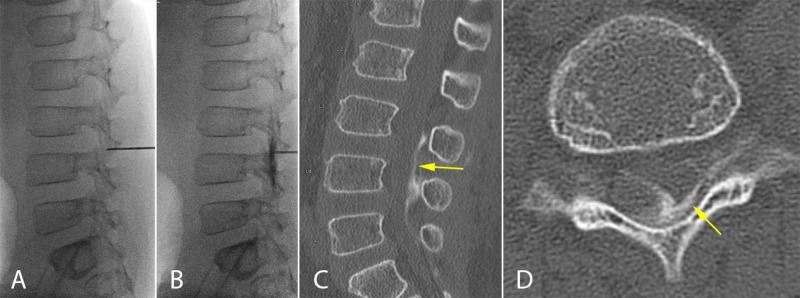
Epidural fibrin injection An example of fluoroscopically guided spinal needle placement (A) and confirmation with contrast (B) intraoperatively before injection of the fibrin sealant in Case 1. Postprocedural CT demonstrates appropriate distribution of the injection (C and D).
